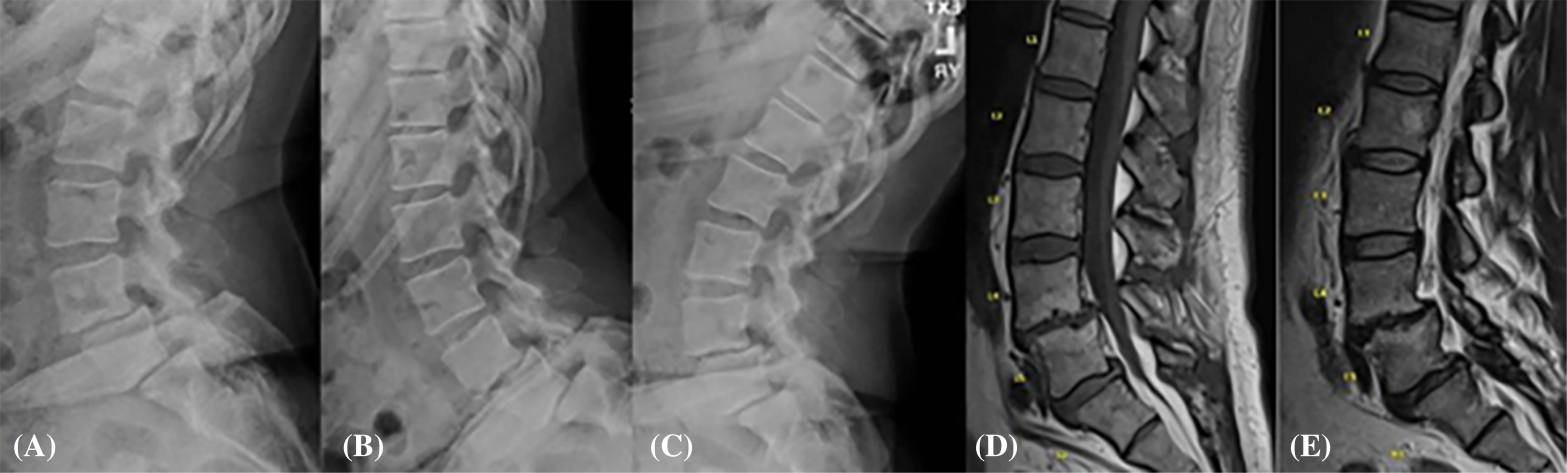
(A)–(C) Dynamic lateral lumbar plain radiographs and (D), (E) lumbar T1‐ and T2‐weighted MRI noting spondylolisthesis at L4‐L5 and L5‐S1 in a 65‐year‐old female.
